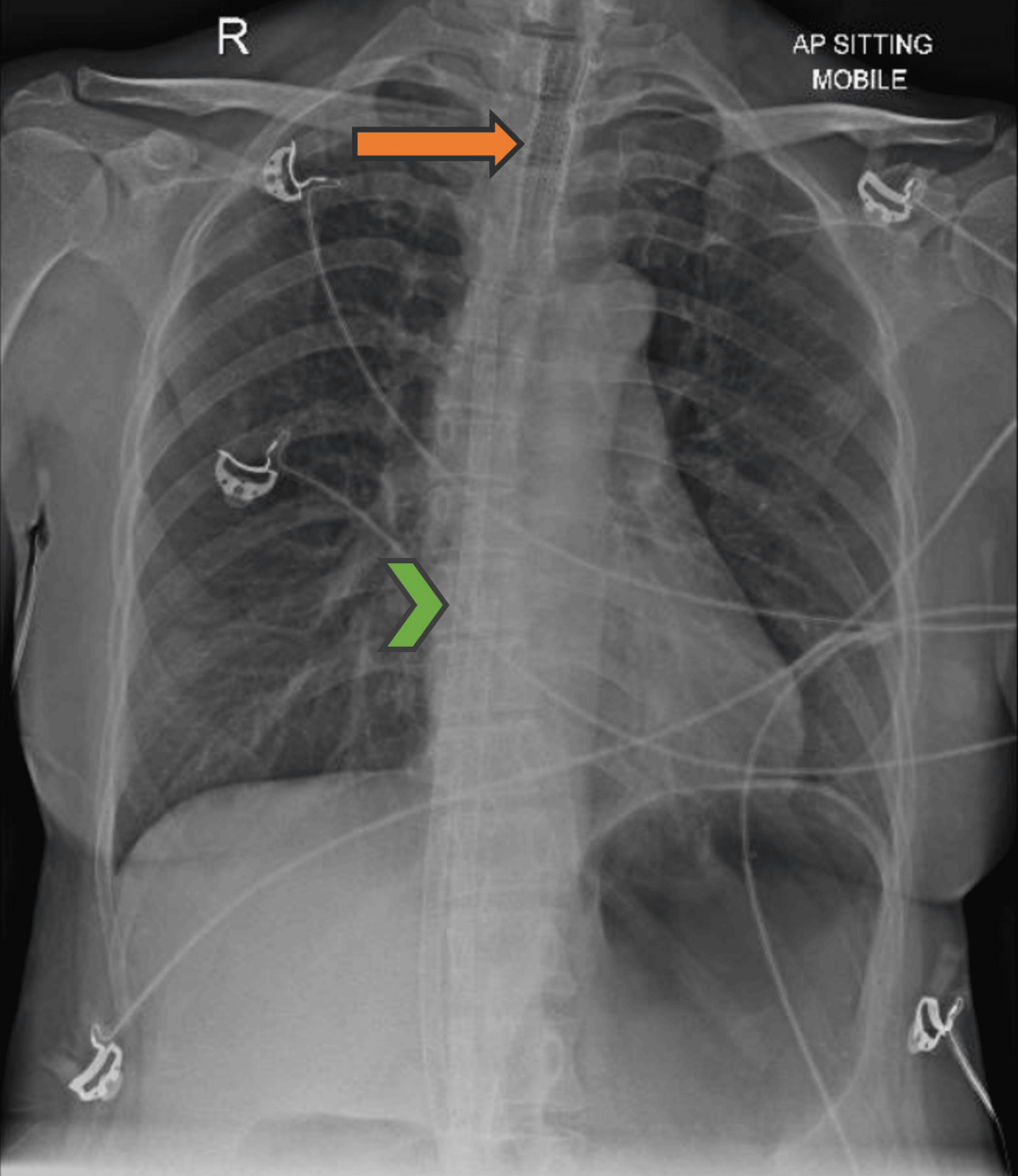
Preoperative chest X-ray showing tracheal stent (orange arrow) and VV-ECMO catheters (green chevron). VV-ECMO, veno-venous extracorporeal membrane oxygenation
